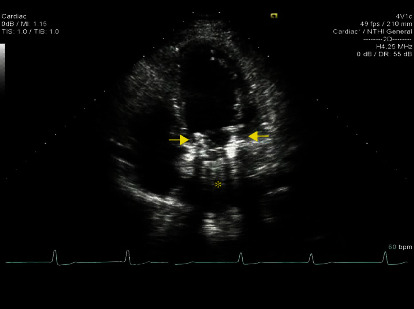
Apical four chamber transthoracic echocardiogram image of the mechanical valve (asterisk) implanted in the failed bioprosthetic valve (arrows).
